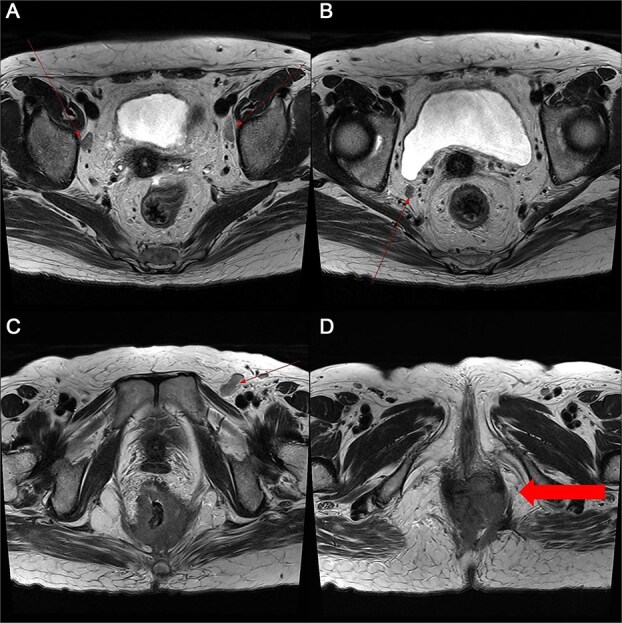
Pelvic MRI demonstrating the progression and extent of perineum and pelvic involvement. (A–C) Indeterminate lymph nodes in the bilateral external iliac chains, right obturator chain, and bilateral inguinal chains (thin arrow). (D) Presence of anorectal cancer with invasion of both levator muscles (thick arrow).
